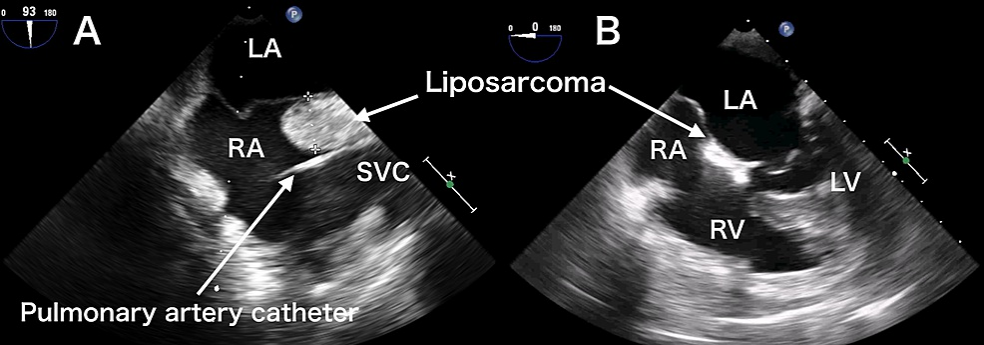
Transesophageal echocardiography during surgery. (A) The bicaval view image showed a finding similar to hypertrophic lipomatous atrial septum, found as a fatty infiltrated structure. (B) The four-chamber view showed that the tumor invaded into the right ventricle. LA: left atrium, LV: left ventricle, RA: right atrium, RV: right ventricle, SVC: superior vena cava.
